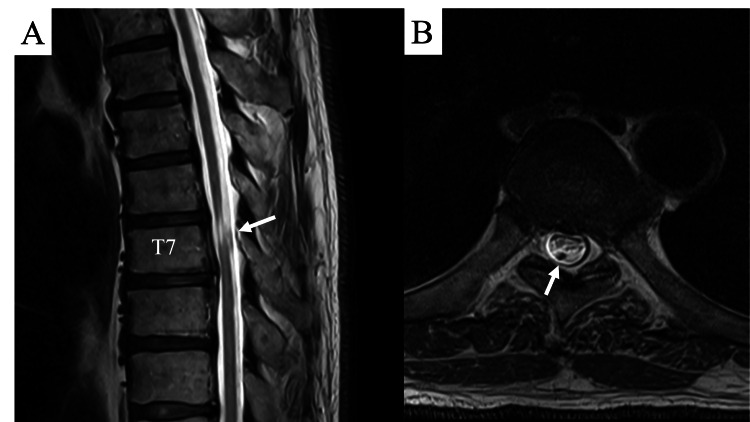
Magnetic resonance images. A: Magnetic resonance image showing atrophy of the spinal cord at the T7 level and a flow void (white arrow) dorsal to the atrophic spinal cord. B: Horizontal slice showed a flattened spinal cord and flow void (white arrow).
